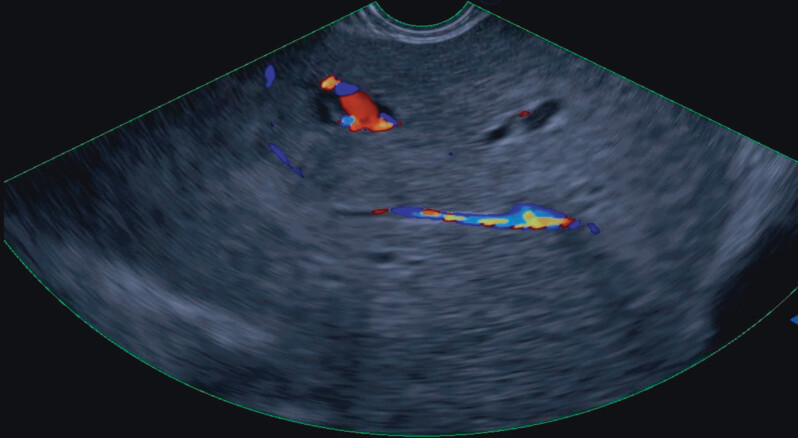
Color Doppler: rhythmic flickering with cardiac motion
